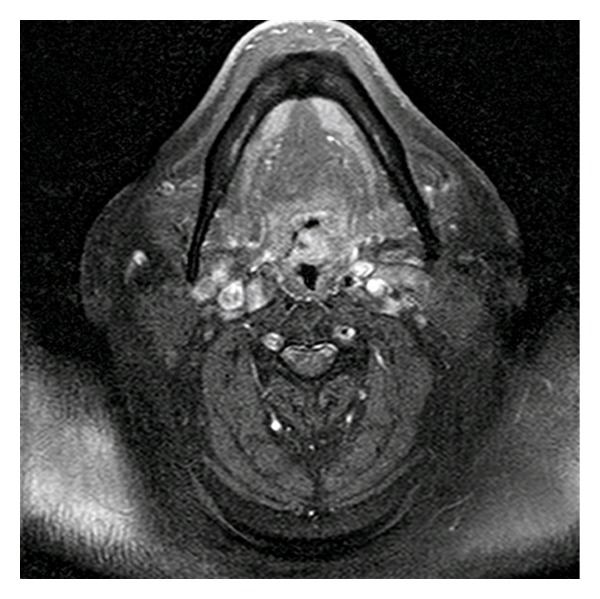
Axial T1 weighted contrast-enhanced MRI image shows left oropharyngeal mass.
